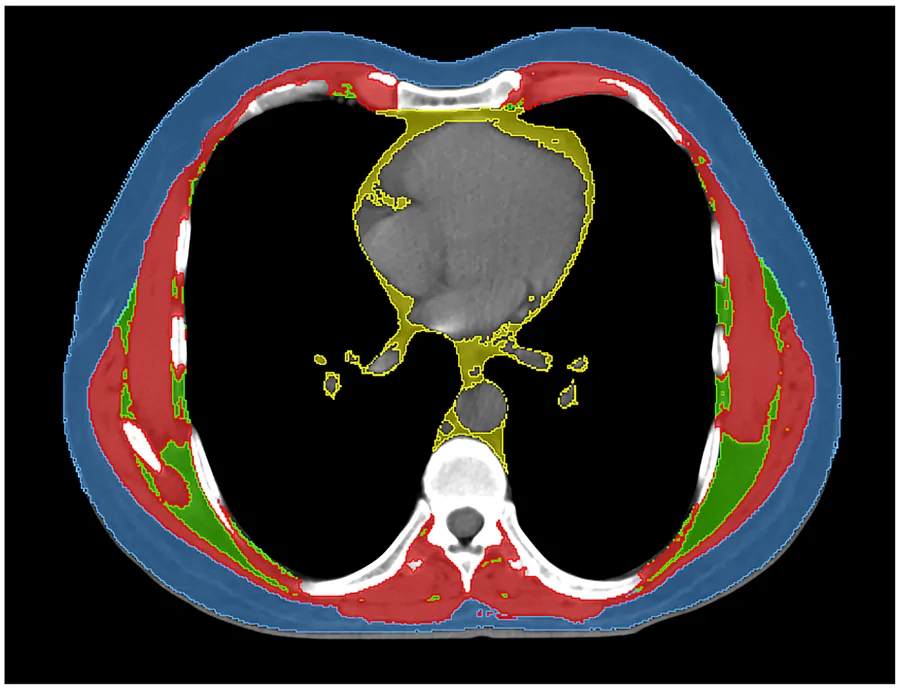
Body composition measurement by computed tomography. Blue indicates SAT, yellow represents VAT, red denotes SM, and green designates IMAT.
